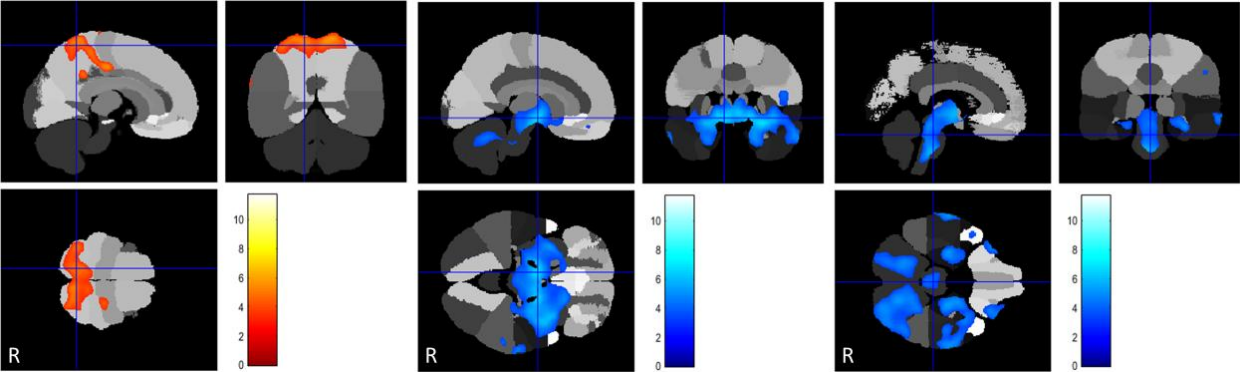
FDG uptake in CP-kernicterus cases (*n* = 13) compared to controls. *Left*: Relative hypermetabolism in superior parietal lobes displayed in a yellow-scale with brighter tones indicating higher *t*-scores. *Middle* and *right*: Areas of relative hypometabolism, including the antero-medial temporal cortex, globi pallidi, thalami, midbrain, pons, dentate-cortical cerebellum and peri-insular cortex, displayed in a blue-scale with brighter tones indicating higher *t*-scores.
